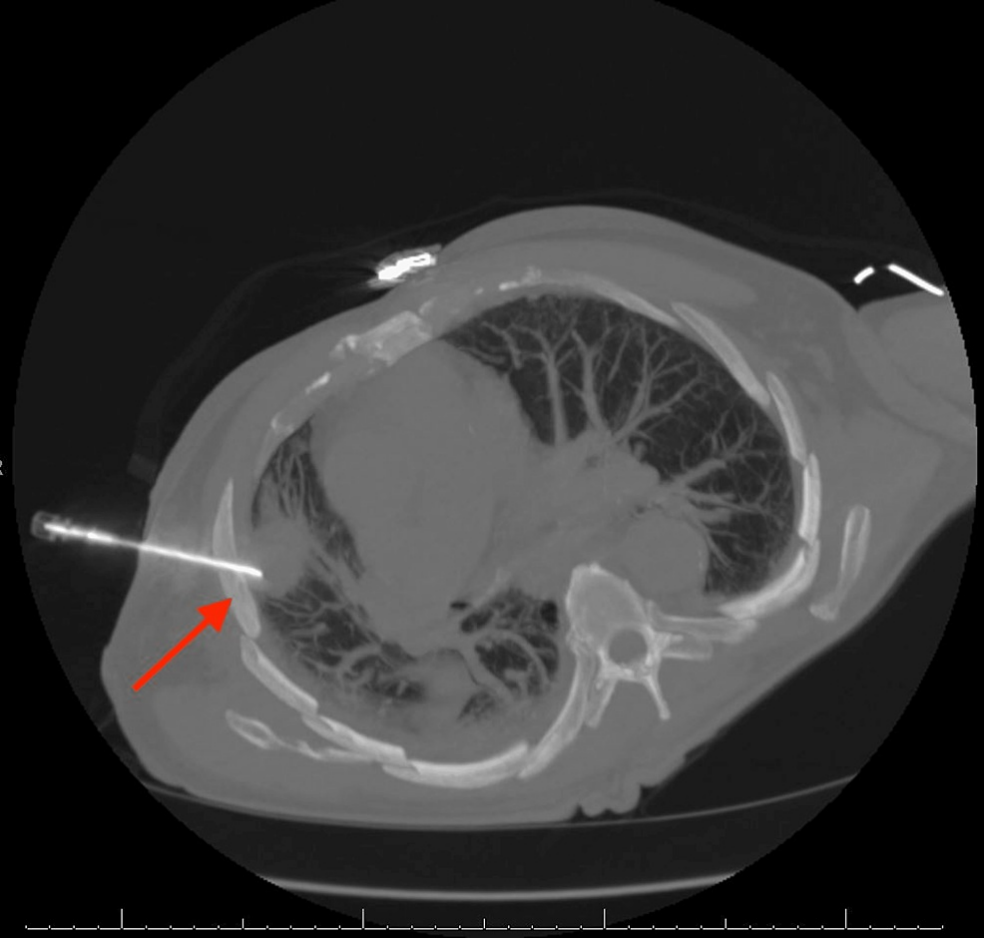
Core needle biopsy (CT-guided) of the right lung mass (arrow).
